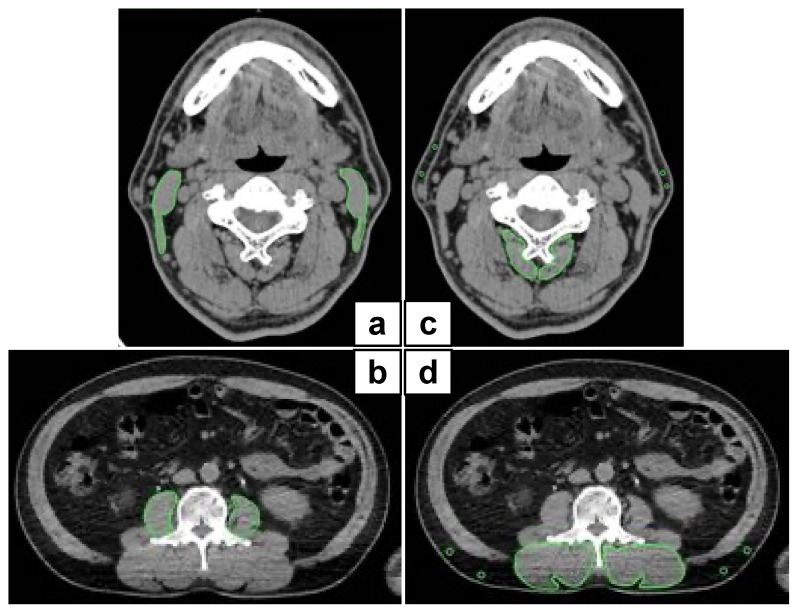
Representative cross-sectional CT images at the C3 and L3 level. (a,b) We precisely traced the subfascial outline of the multifidus and sternocleidomastoid muscles. The SCMI and PMI were determined by normalizing the cross-sectional areas to height (cm^2^/m^2^); (c,d) P-IMAC and IMAC were measured by dividing the CT values of bilateral multifidus muscles at the C3 and L3 levels by the CT attenuation values of subcutaneous fat, respectively. CT, computed tomography; SCMI, sternocleidomastoid muscle mass index; PMI, psoas muscle index; P-IMAC, processus spinosus muscle—intramuscular adipose tissue content; IMAC, intramuscular adipose tissue content.
